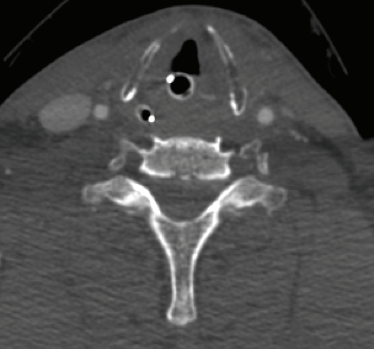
Neck CT angiogram axial-image shows complete occlusion of bilateral VA. Note the absence of contrast dye within the foramen transversarium bilaterally whereas bilateral carotid arteries are contrast filled.
